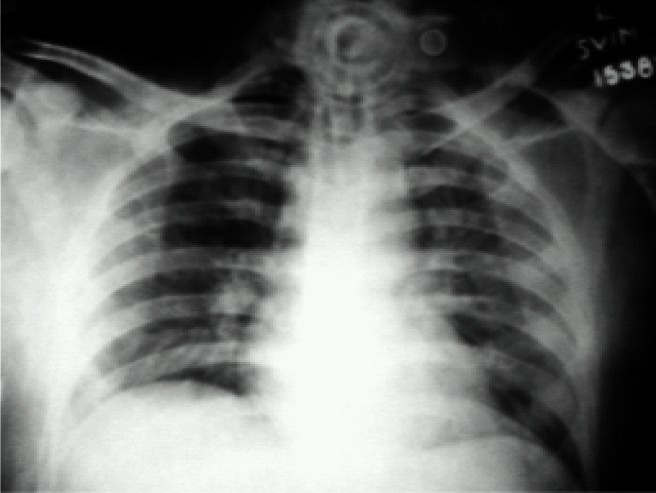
Chest X-ray on 14th day showing clearing of lung fields
